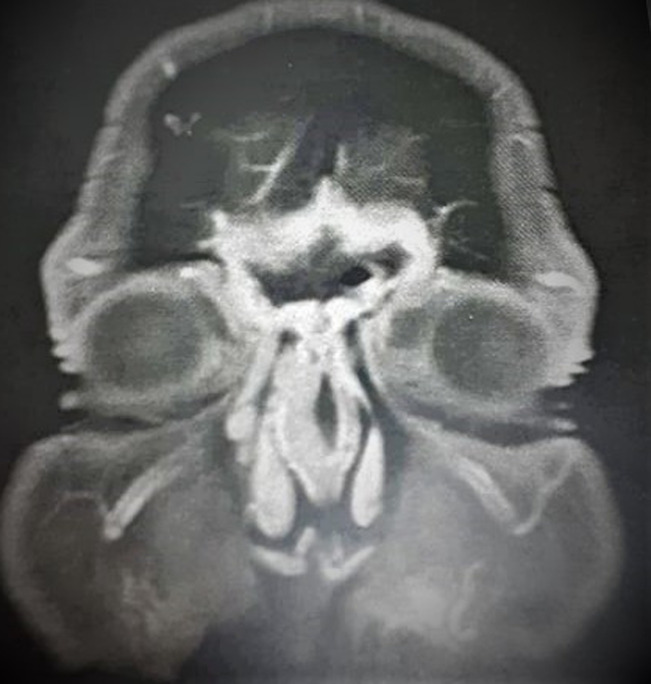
cerebral magnetic resonance imaging showing a bifrontal meningeal contrast enhancement without intracranial invasion
